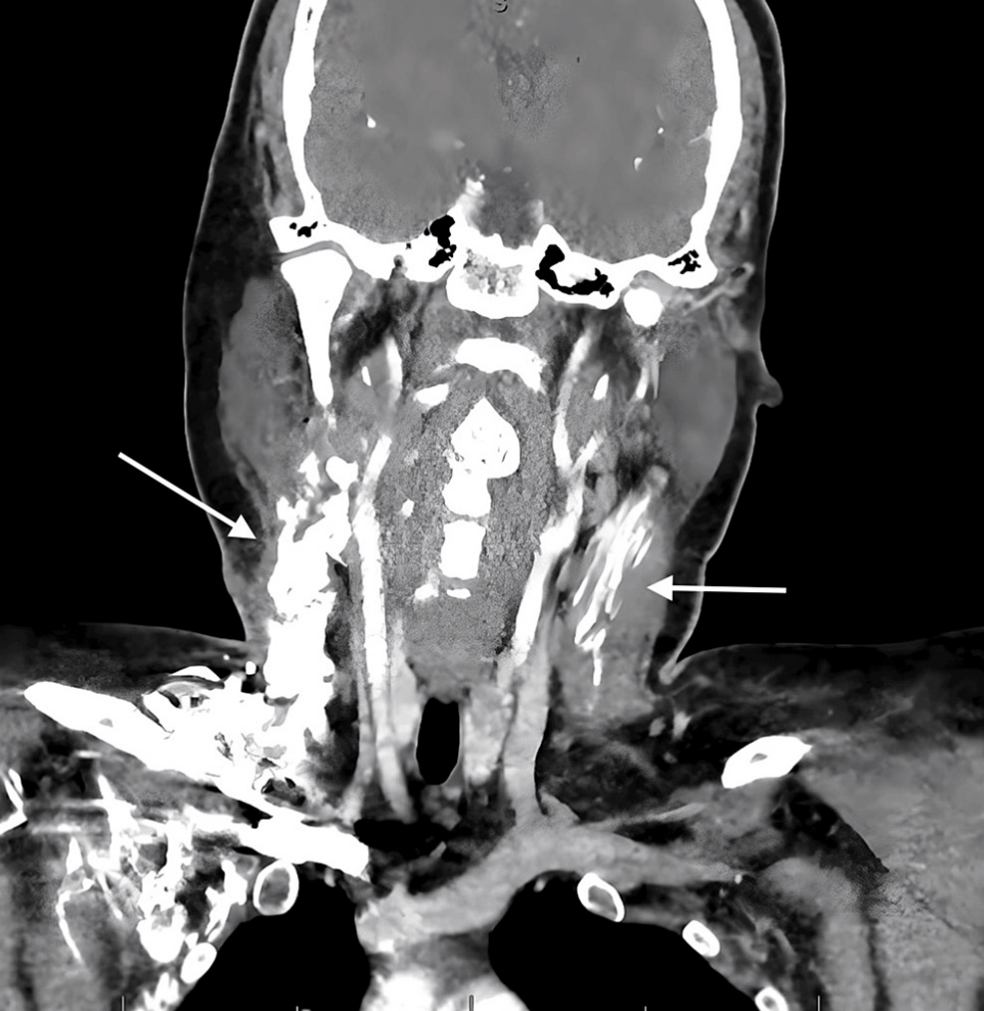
CT scan of the neck with heterotropic bone formation in bilateral sternocleidomastoid muscle
